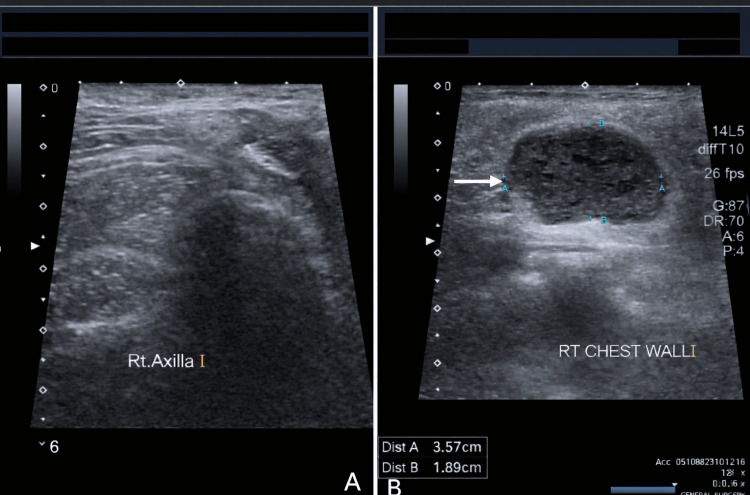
Ultrasonogram images of the right axilla (A), showing a clear axilla with no lymph nodes, and the right chest wall tumor (B), indicated by a white arrow, demonstrating well-defined borders without areas of calcification, measuring 3.57 cm × 1.86 cm
